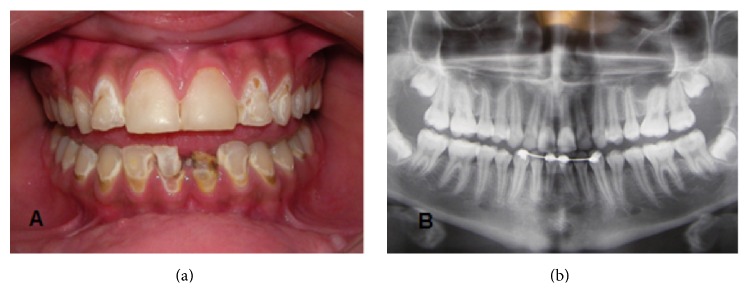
(a) The initial clinical appearance demonstrating carious lesions on buccal regions; (b) radiographic examination showing the fixed lingual orthodontic appliance for treatment contention and carious lesions.
